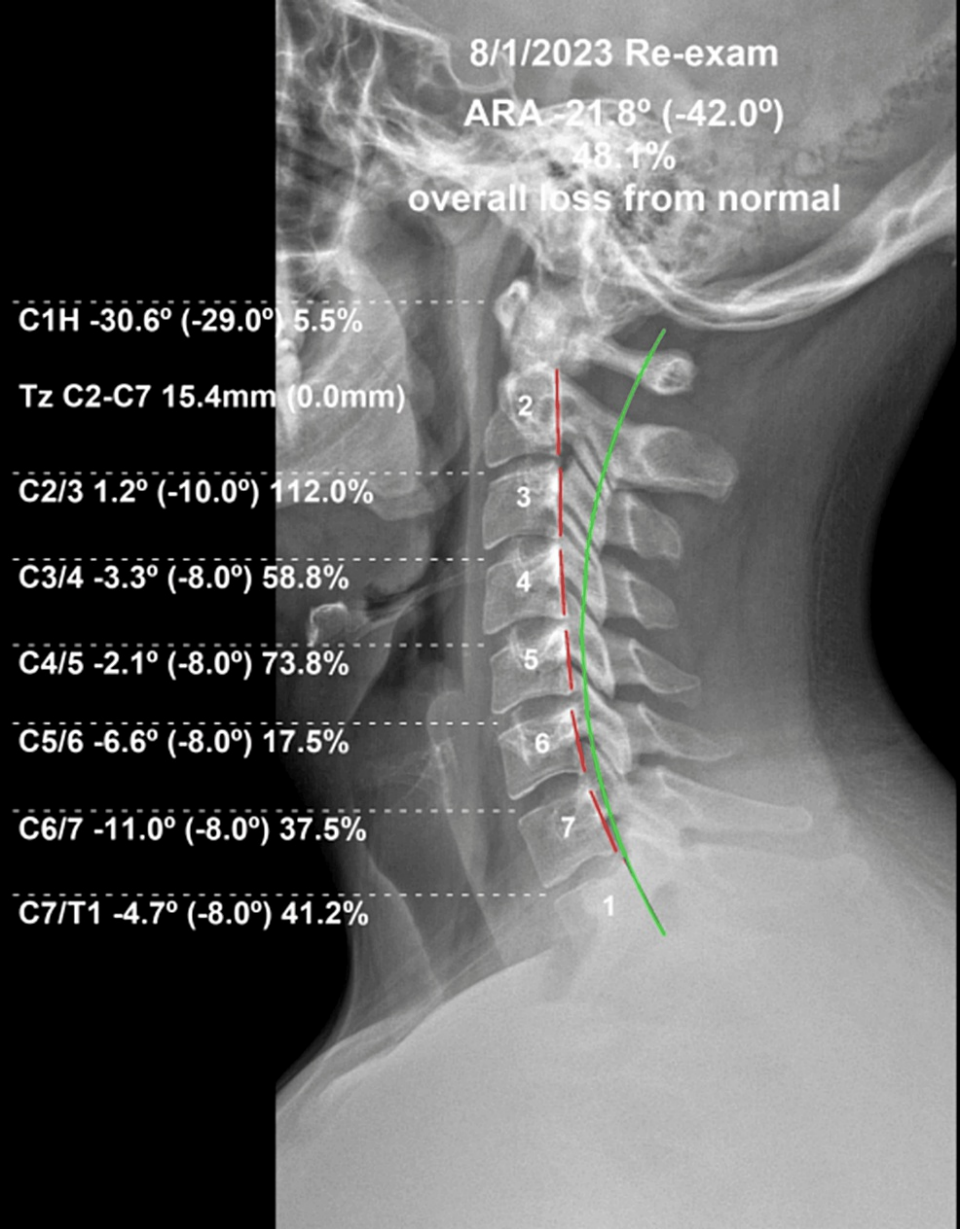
Ten-month follow-up lateral cervical radiograph Long-term lateral cervical radiograph demonstrates sagittal balance is well maintained and the lordosis is stable with a regimen of one in-office treatment per month and consistent home Denneroll® cervical orthotic use. The red line is the patient's posterior tangents, and the green line represents an ideal mathematical lordotic model
